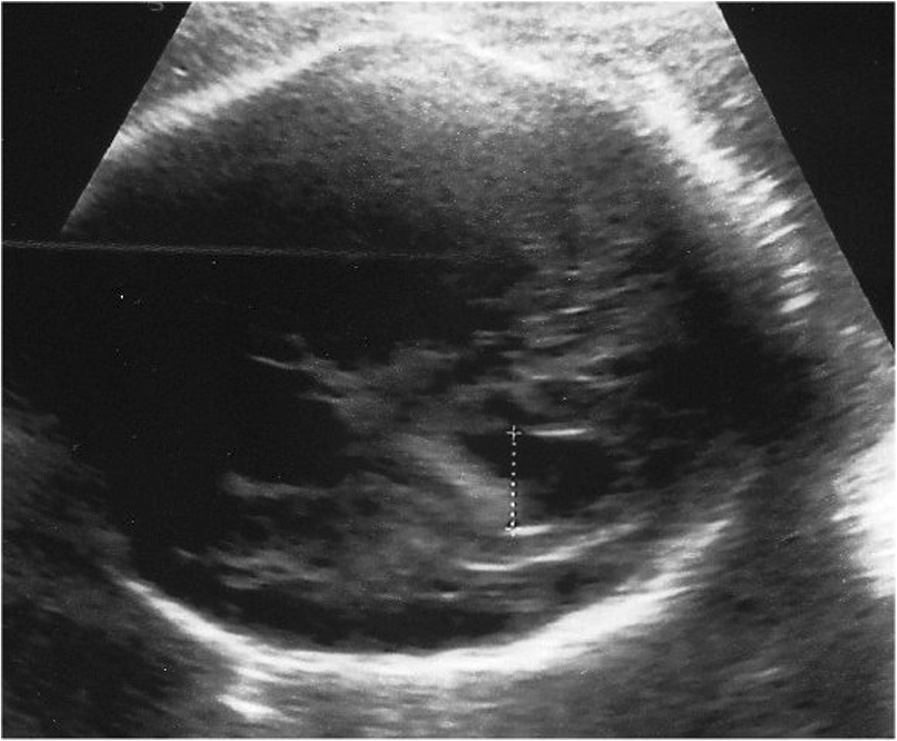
Prenatal ultrasonographic image from our patient at 32 + 3 GW. Left ventriculomegaly.
